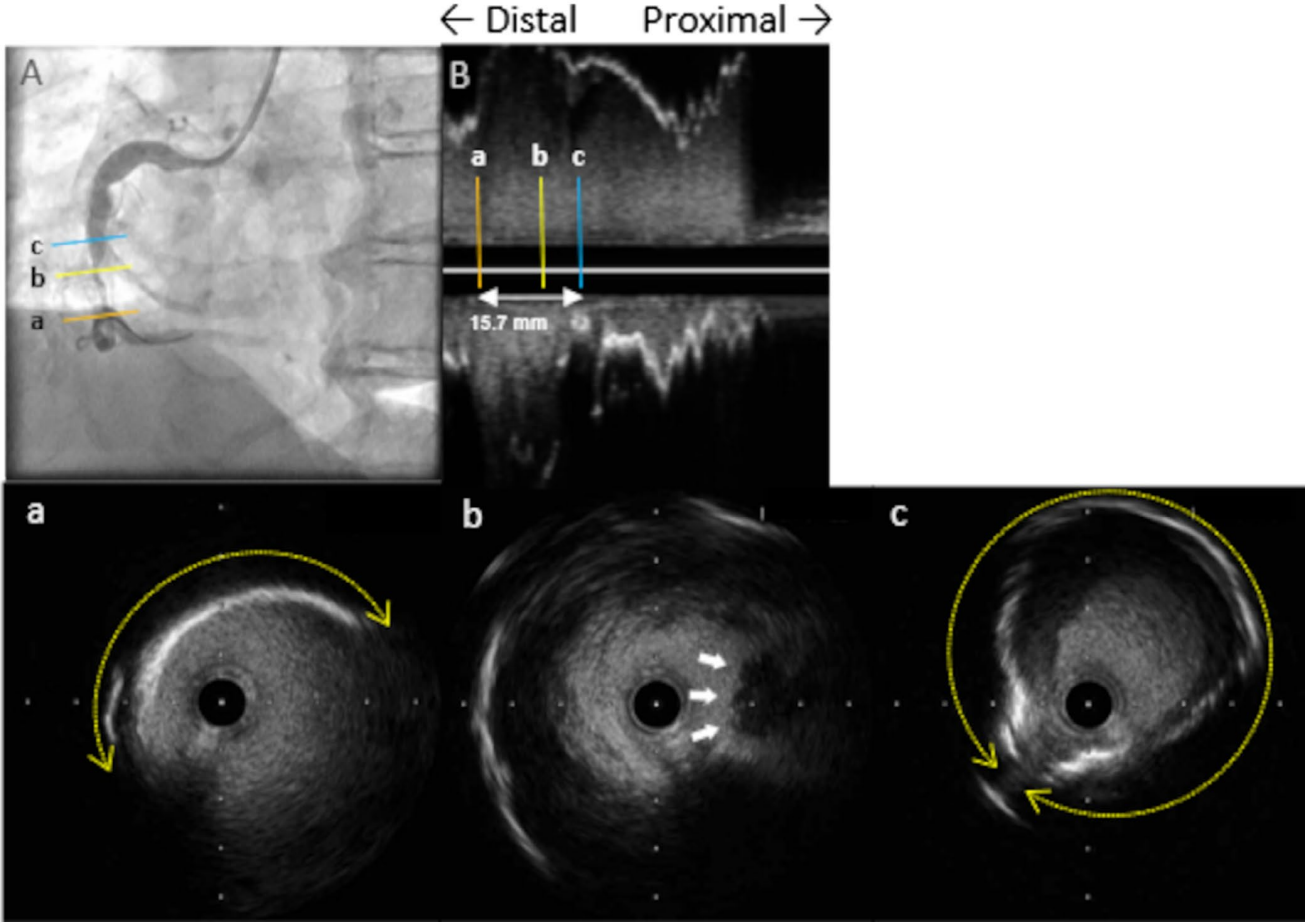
Angiographic and intravascular ultrasound (IVUS) imaging of the right coronary artery after recanalization, showed aneurysm, calcification, and thrombus formation. (A) Right coronary angiography after recanalization. (B) Longitudinal intravascular ultrasound (IVUS) images demonstrated a lesion measuring 15.7 mm in length (white arrow). Each of the a, b, and c lines indicated a cross‐sectional IVUS image. (a) At the distal segment, IVUS showed an aneurysm and 180° calcification (yellow dotted circle). (b) Thrombus formation (white arrows) at the culprit lesion, with a lumen diameter of 2.45 × 2.87 mm and a minimum lumen area of 5.47 mm^2^. (c) At the proximal segment, the vessel exhibited protrusion outward and 360° vessel wall calcification (yellow dotted circle).
